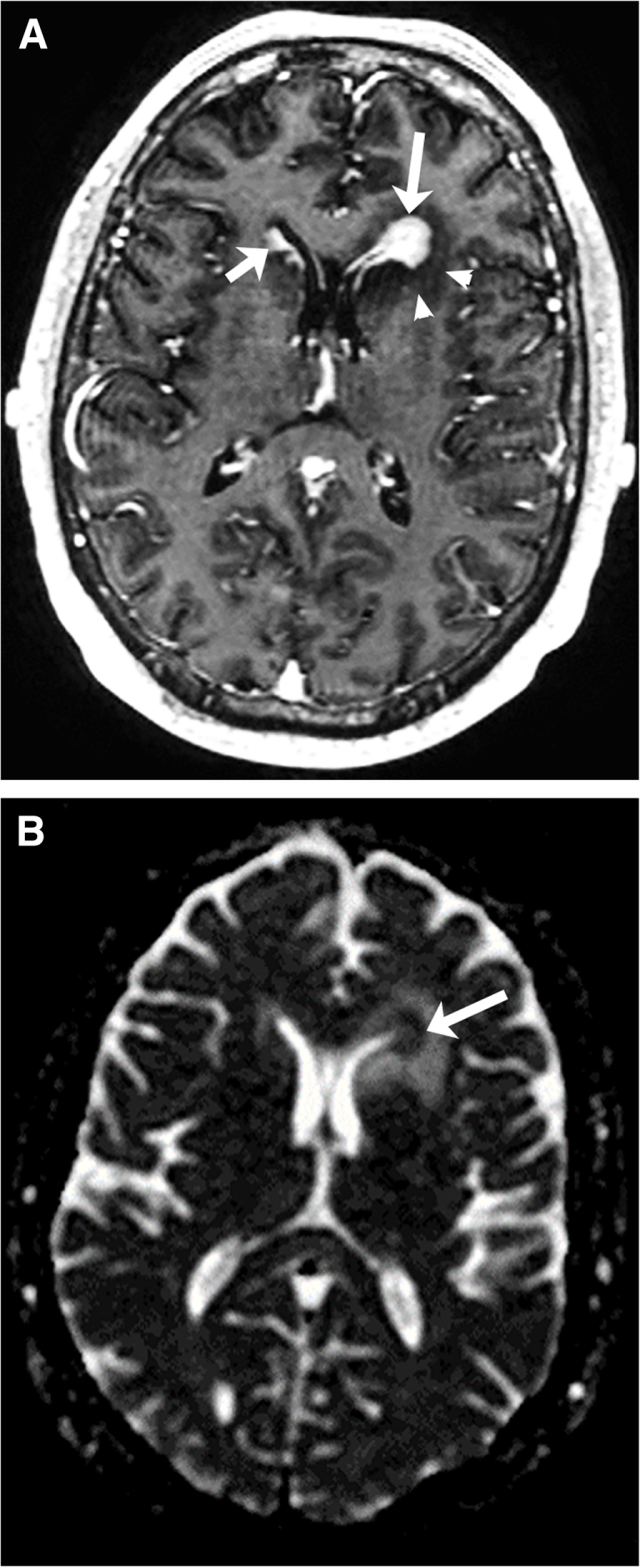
Primary central nervous system lymphoma. T1-weighted image with gadolinium (a) shows a diffusely enhanced lesion (long arrow) in the periventricular left frontal horn with mild surrounding vasogenic edema (arrowheads). Also, note the ependymal enhancement along the right frontal horn (short arrow). ADC map (b) shows homogeneous diffusion restriction (arrow)
